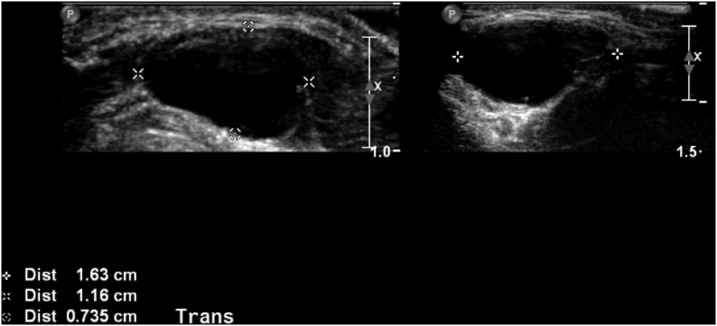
An ultrasound scan of the anterior midline neck mass. A well-defined 16 × 12 × 7 mm avascular thin walled cystic structure is demonstrated.
